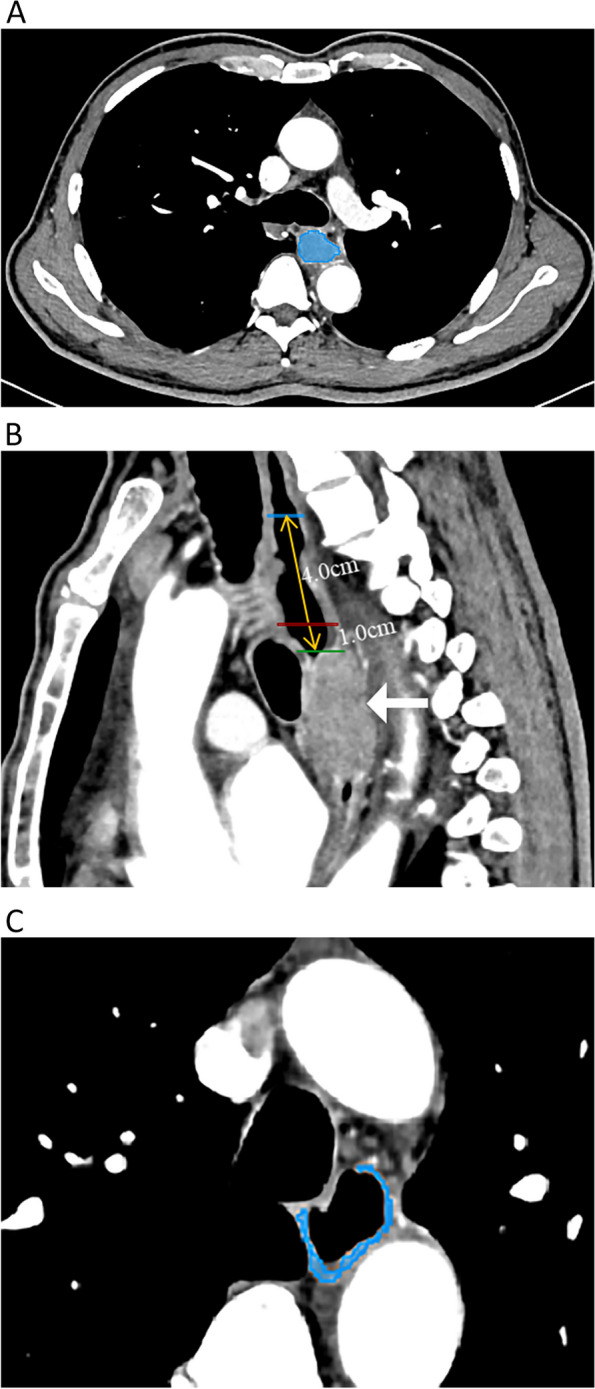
In a 64-year-old male patient with esophageal squamous cell carcinoma (ESCC), the region of interest (ROI) was delineated slice by slice on axial contrast-enhanced computed tomography (CECT) images (A). The white arrow refers to the primary tumor of ESCC; the blue, red and green solid lines represent the position lines of the proximal tumor-distant (PTD) tissue, proximal tumor-adjacent (PTA) tissue and the proximal margin of ESCC on the reconstructed sagittal CECT image, respectively; and the yellow arrow along the longitudinal axis of the esophagus indicates the ruler measuring line for defining the boundary lines of the PTD and PTA tissues (B). The ROI covering more than half of the esophageal wall for PTA tissue is manually drawn on an axial magnified CECT image (C). The ROI delineation of PTD tissue is performed similarly to that of PTA tissue
